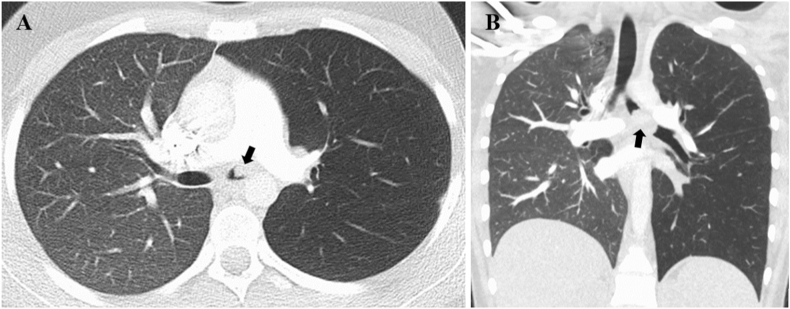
Chest CT for case 4. A) Axial and B) Coronal images demonstrated a 2.5 cm occlusive lesion within the left mainstem bronchus (black arrow) with resultant air trapping on the left side.
